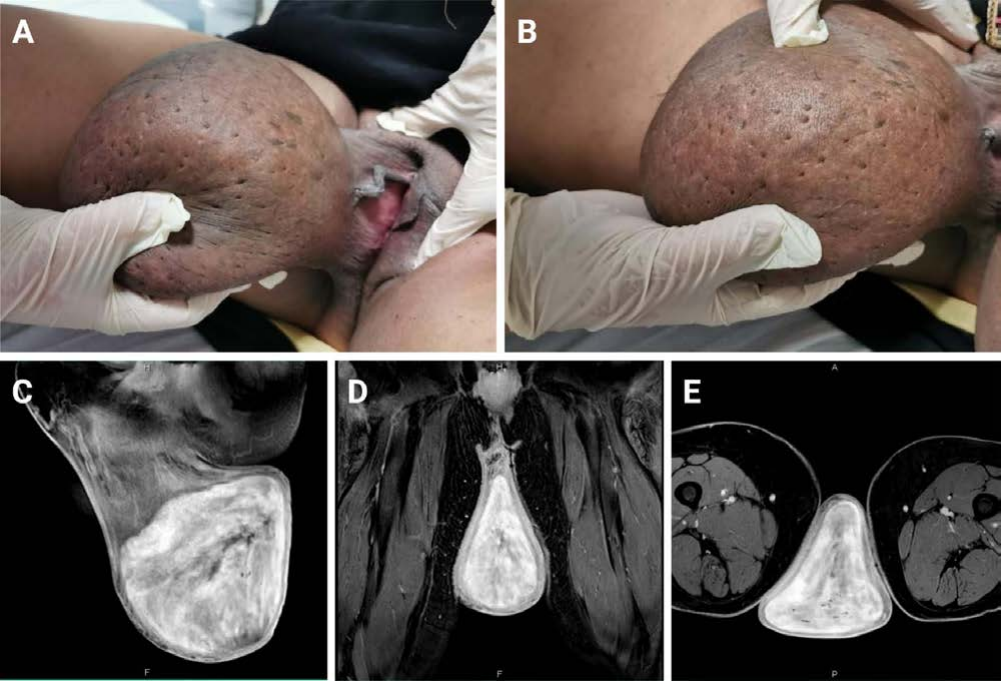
A right-sided large tender vulvar mass with normally looking vagina (A, B). Pelvic MRI with contrast revealing a large heterogeneously enhancing soft tissue mass, measuring 13.1 × 10.9 × 10.7 cm expending the left vulva with internal and peripheral voids resembling feeding vessels. MRI = magnetic resonance imaging.
